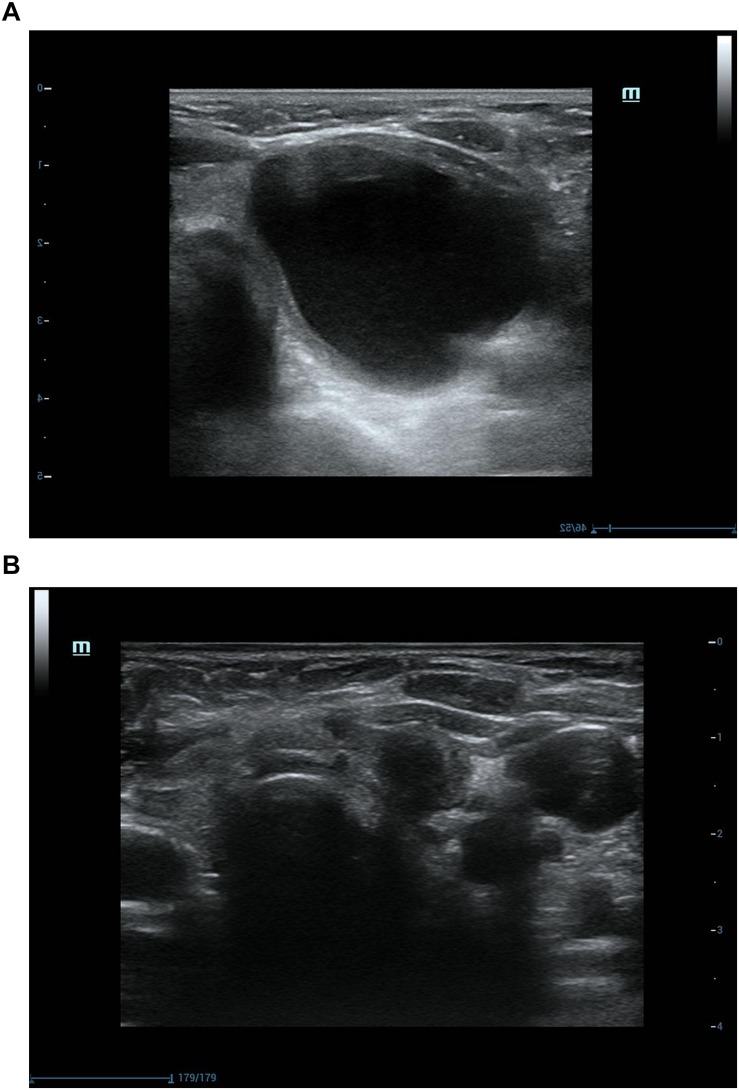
Ultrasonograpic image of a 70 years old female before and after treatment.(A) A purely cystic nodule of sonography before USG-LIA.(B) 18 months after USG-LIA.
